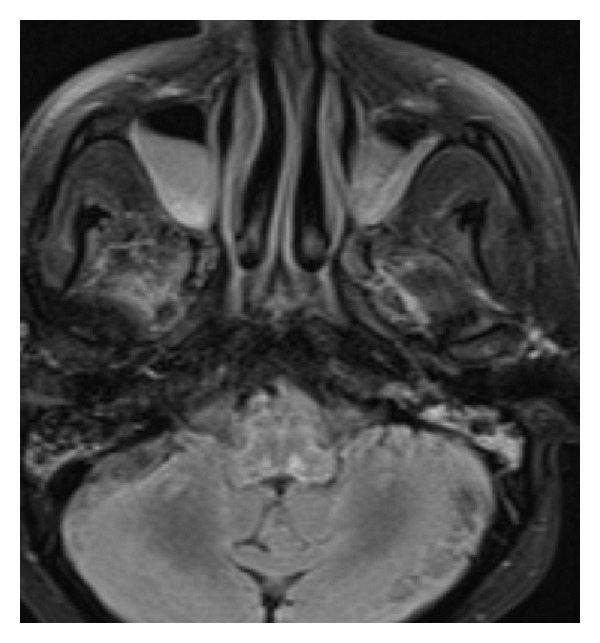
MRI brain showing increased T2 FLAIR signal in brainstem.
